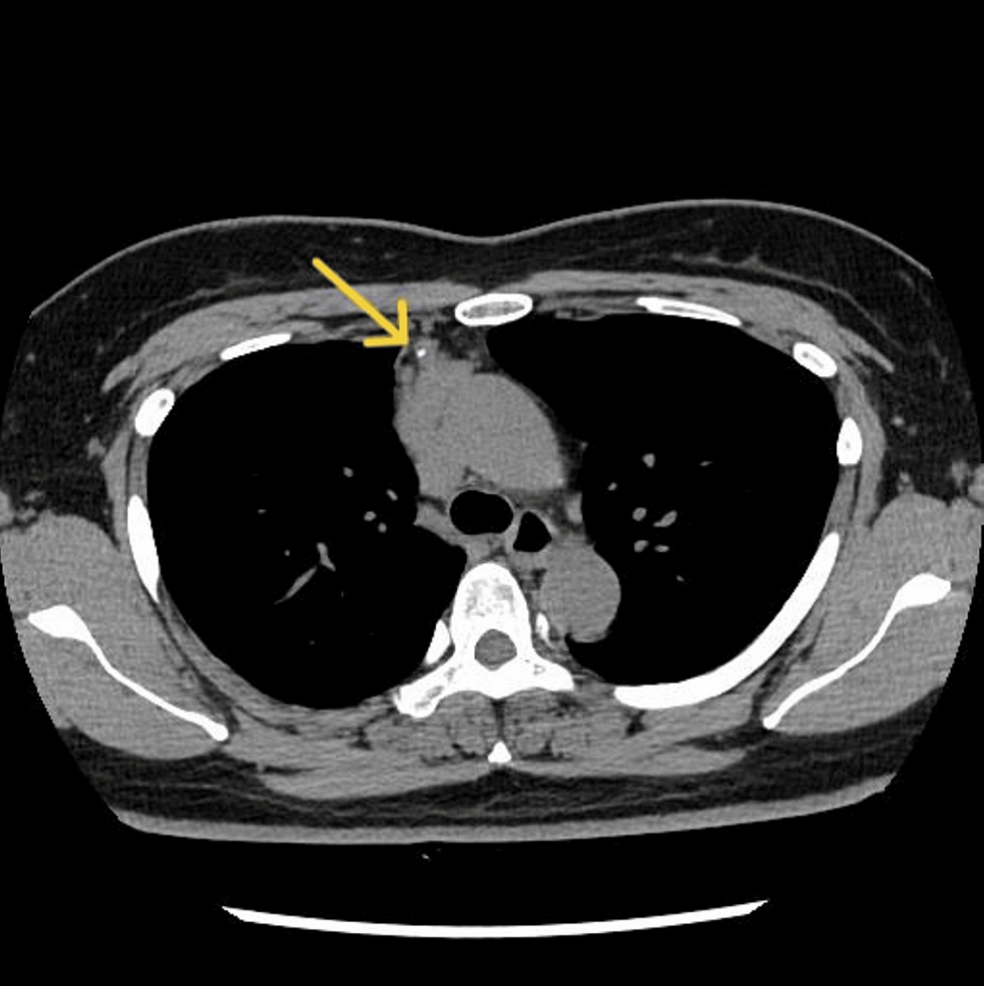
Unenhanced compyted tomography (CT) showing a lobulated mass with sparse calcification in the anterior mediastinum.
